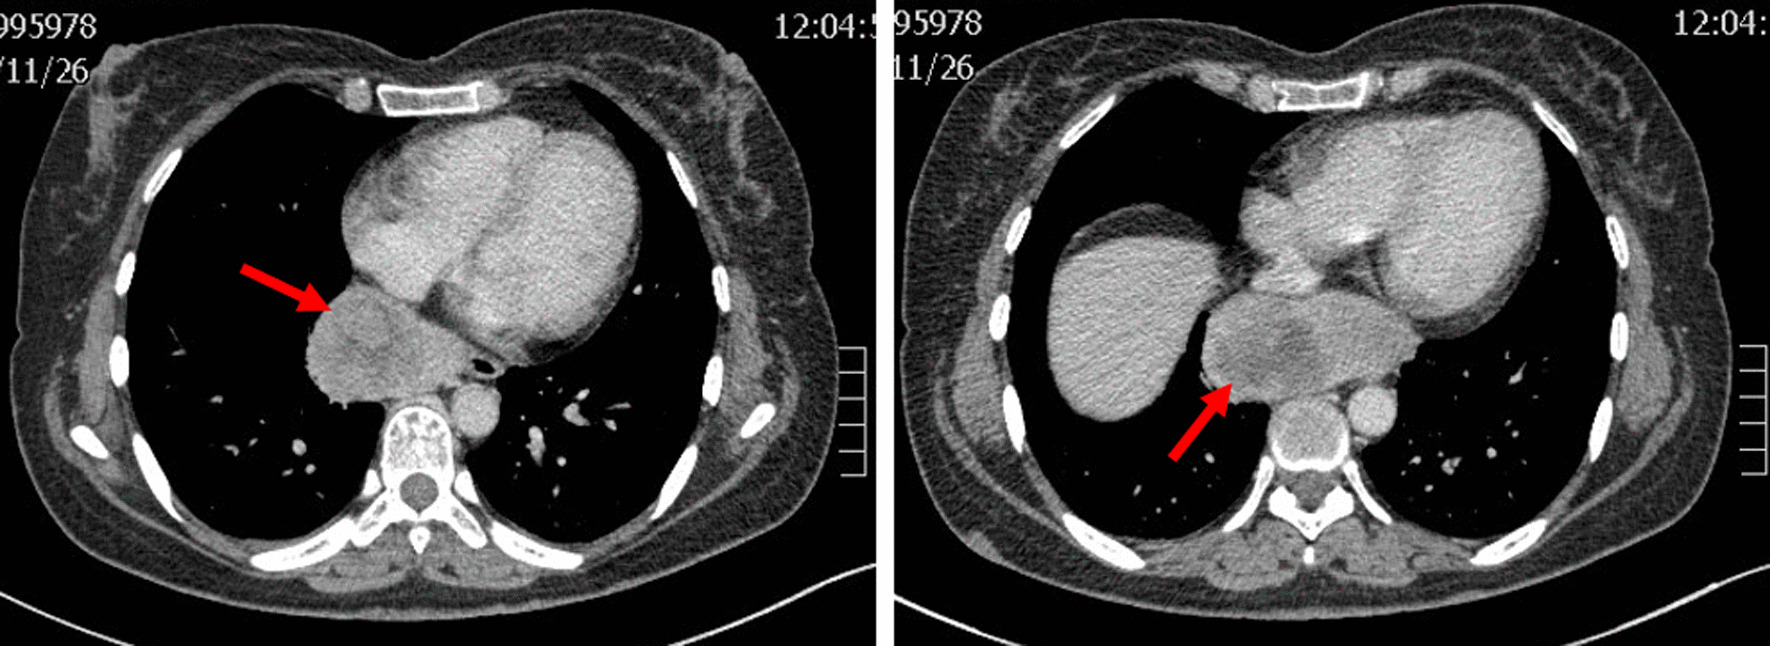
Chest enhanced CT showing a round soft tissue density sized 8.4 × 4.0 cm (red arrow)
